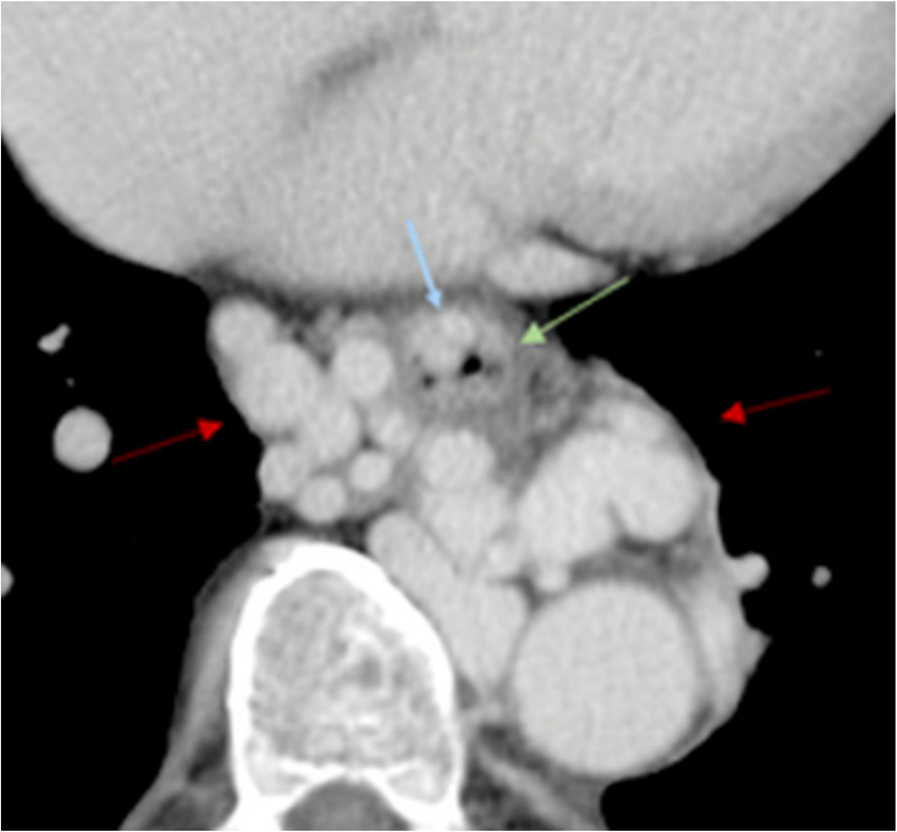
Esophageal and paraesophageal varices. Portal venous phase CT axial images show paraesophageal varices (red arrow) in the mediastinum, appearing as dilated tortuous veins surrounding the outer surface of the esophageal inferior third (green arrow), and esophageal varices (blue arrow), presenting as submucosal varicose veins within the esophagus wall
